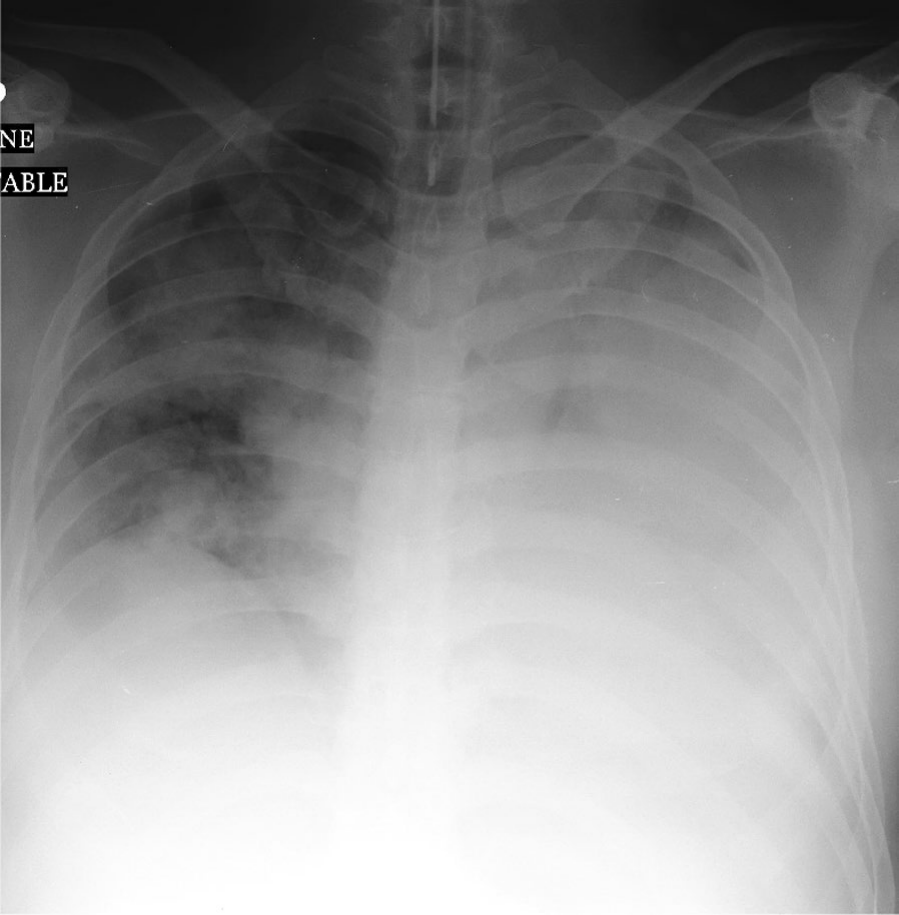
A 52-year-old man who developed Middle East respiratory syndrome coronavirus. Frontal chest radiograph obtained on day 8 shows bilateral multifocal patchy airspace disease with predominant hemiopacification of the left lung; chest radiographic score is 14 with LDH of 760. The patient died ten days after initial admission
